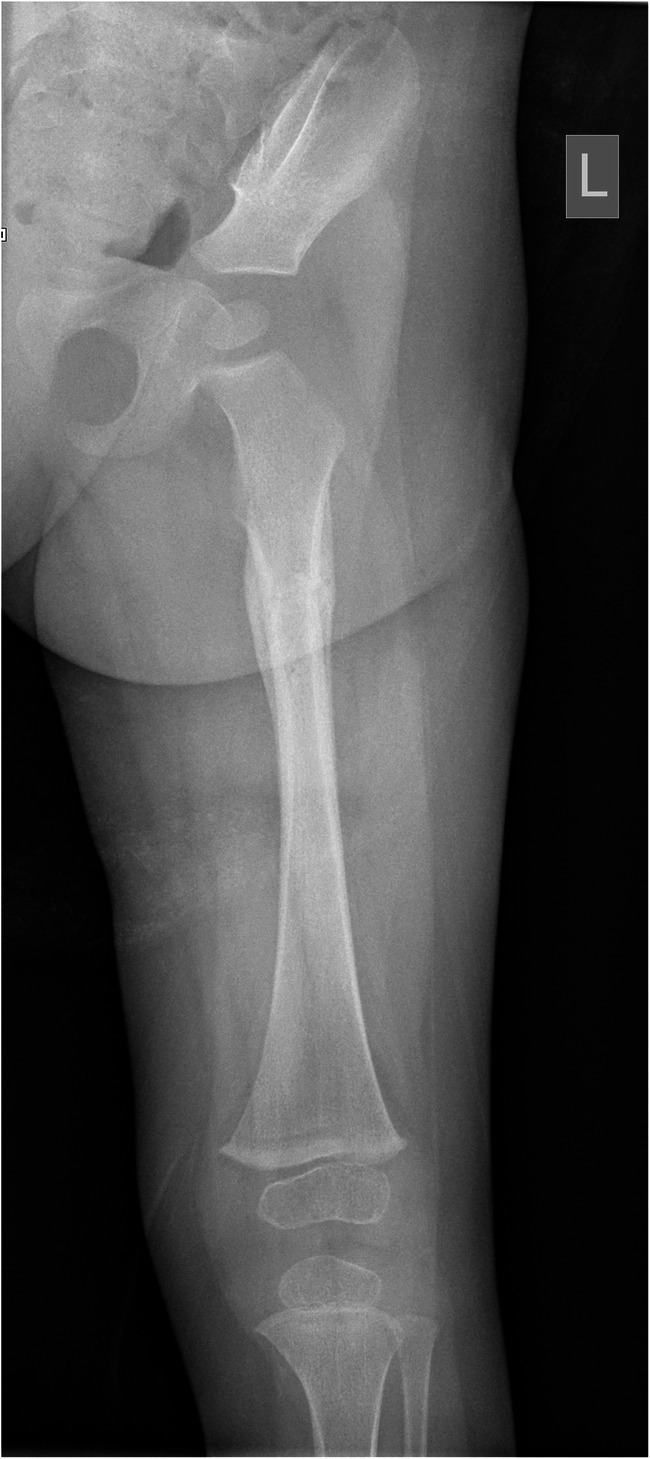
An anteroposterior radiograph of the left femur in a 15-month-old girl shows callus
